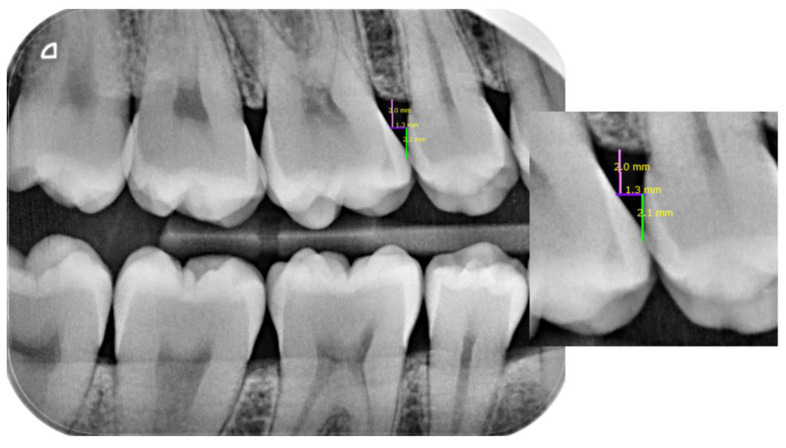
Measurements on radiography with color lines. Pink line: 2 mm height from the bone crest. Purple line: cervical embrasure measurement in mesio-distal direction at 2 mm from the bone crest (width of the wedge). Green line: distance between the ideal contact area to 2 mm from the bone crest (height of the wedge).
